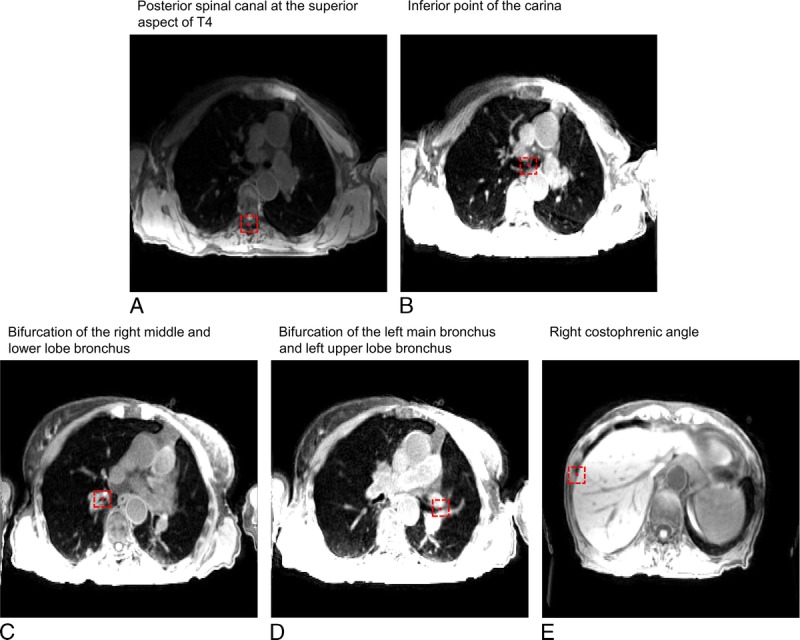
An example of control point delineation for patient 8 on a snapshot image of 4D-T1w MRI. The control points (red circles) are contained within dashed red boxes to assist visualization. The presented windowing scheme was optimized by the radiation oncologist for viewing of the anatomical landmark and not for surrounding anatomy.
